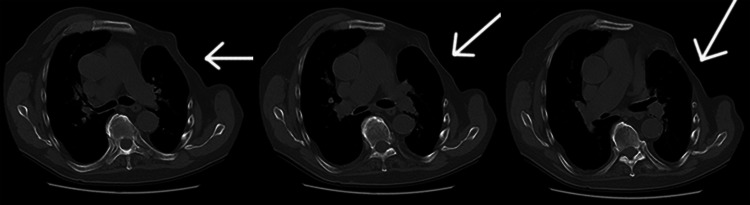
Non-contrast CT chest (axial view) The arrows show a complete absence of the left pectoralis major muscle and the rudimentary pectoralis minor muscle.
